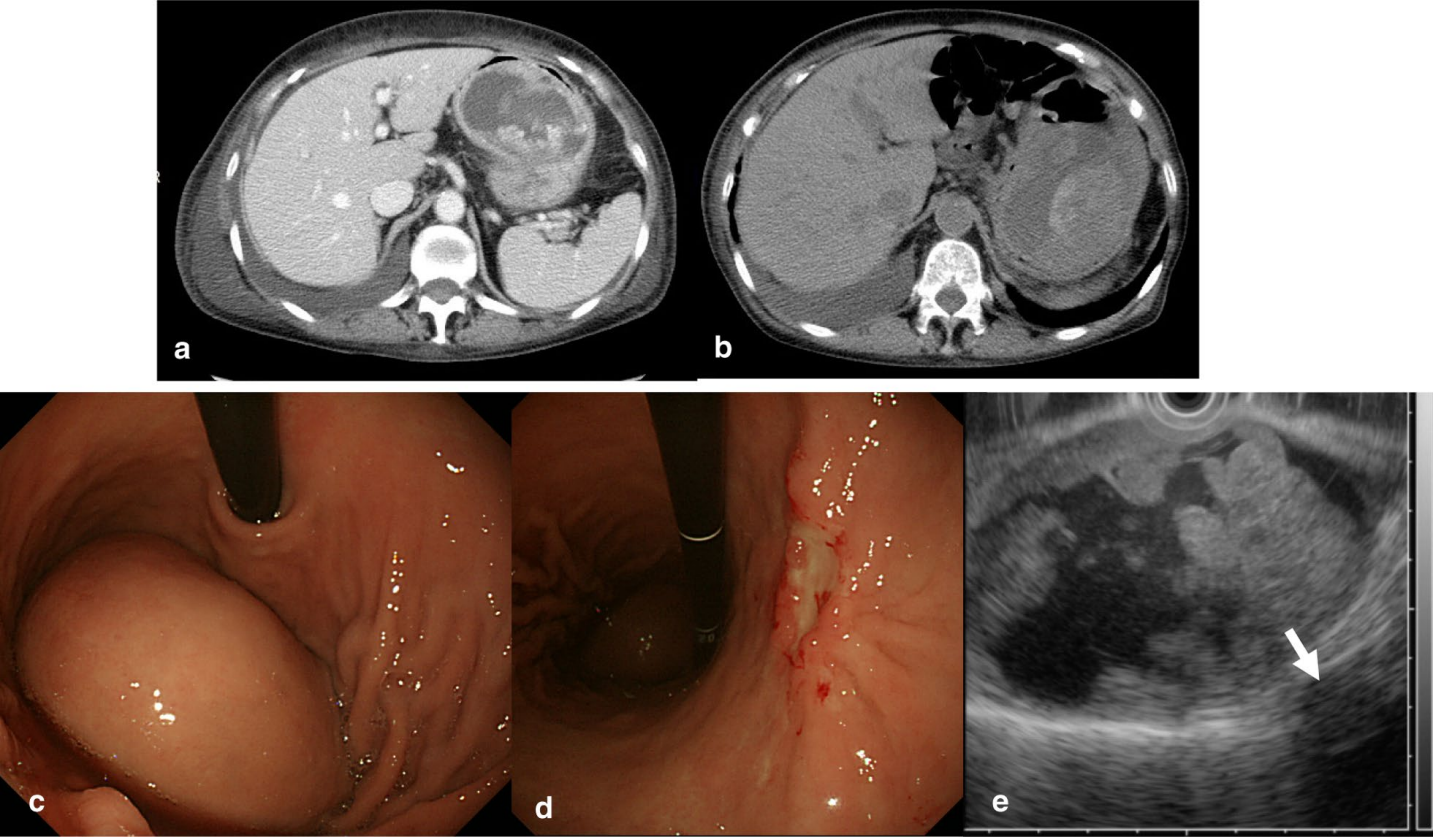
a On admission contrast-enhanced CT showed a mass of 60 × 80 mm in diameter at the fornix of the stomach, which had intra-tumor heterogeneity with enhanced margin. Intra-tumoral extravasation of contrast agent was observed. b Twelve days after admission plain CT showed that the tumor grew to 65 × 90 mm in diameter. c On endoscopy, a submucosal bulge was identified with overlying normal mucosa on the anterior wall at the fornix of the stomach. d A 0–IIc ulcerative lesion surrounded by abnormal edge of converging folds, namely club-like thickening, was perceived at the lesser curvature of the gastric angle. e Endoscopic ultrasound demonstrated that the subepithelial cystic tumor containing hypoechoic papillary component arising from the 4th layer of the gastric wall, muscularis propria (arrow)
